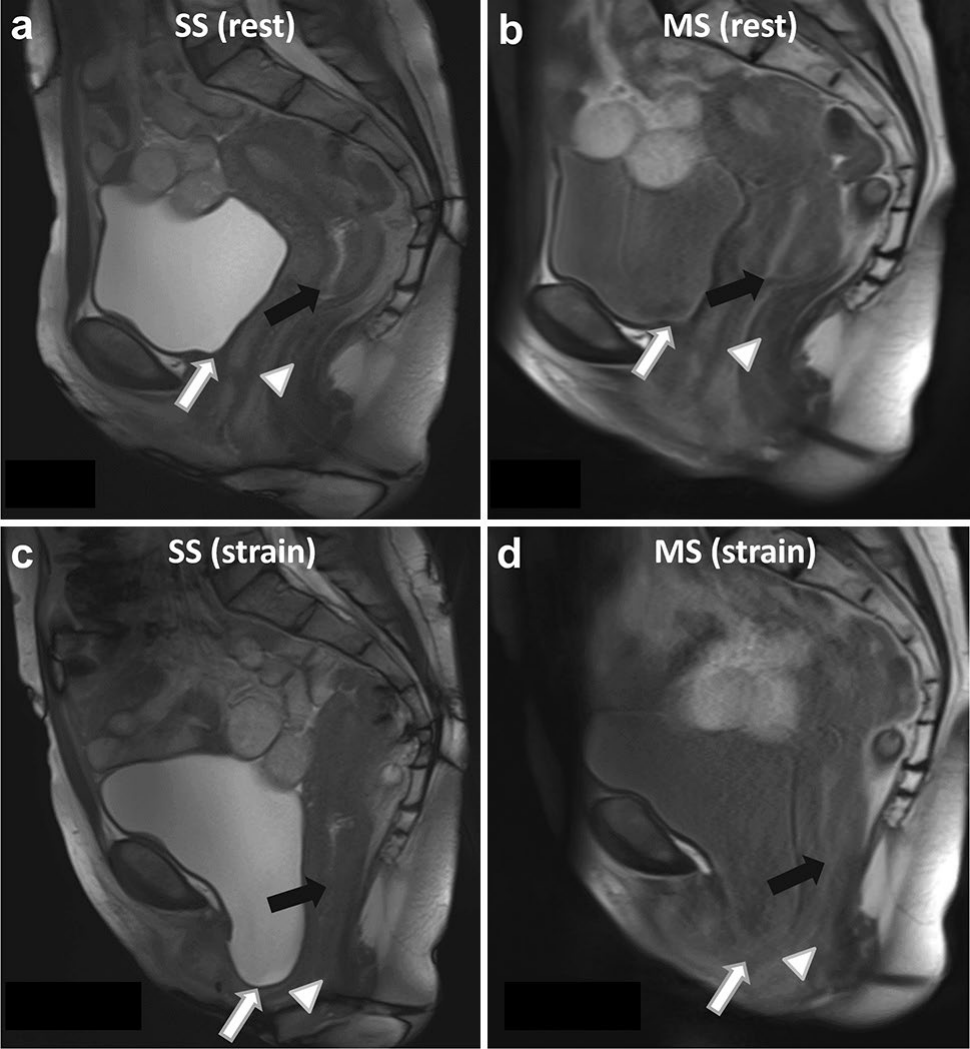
38-year old primiparous woman with symptomatic pelvic organ prolapse in involving all three compartments and a POP-Q score of III. Sagittal single-slice (a, c) and multi-slice (b, d) MR images at rest (a, b) and maximum straining (c, d) are shown. The bladder (white arrow), cervix (black arrow) and anorectal junction (white arrowhead) are annotated. At rest, the bladder point was 1.6 cm and 1.6 cm, the cervix point was 4.1 cm and 3.7 cm, and the anorectal junction was 1.4 cm and 1.0 cm above the PICS line in the single-slice and multi-slice sequences, respectively. During maximum straining, the bladder point was 4.1 cm and 3.4 cm below, the cervix point was 0.2 cm and 0.0 cm above, and the anorectal junction was 3.1 cm and 3.1 cm below the PICS line in the single-slice and multi-slice sequence, respectively. Differences between single and multi-slice sequences were small (0.1–0.4 cm at rest and 0.0–0.7 cm at straining) and there was no difference in the POP grading between the single-slice and multi-slice sequence (grade 2 descent of the anterior compartment, and grade 1 descent of the posterior compartment)
